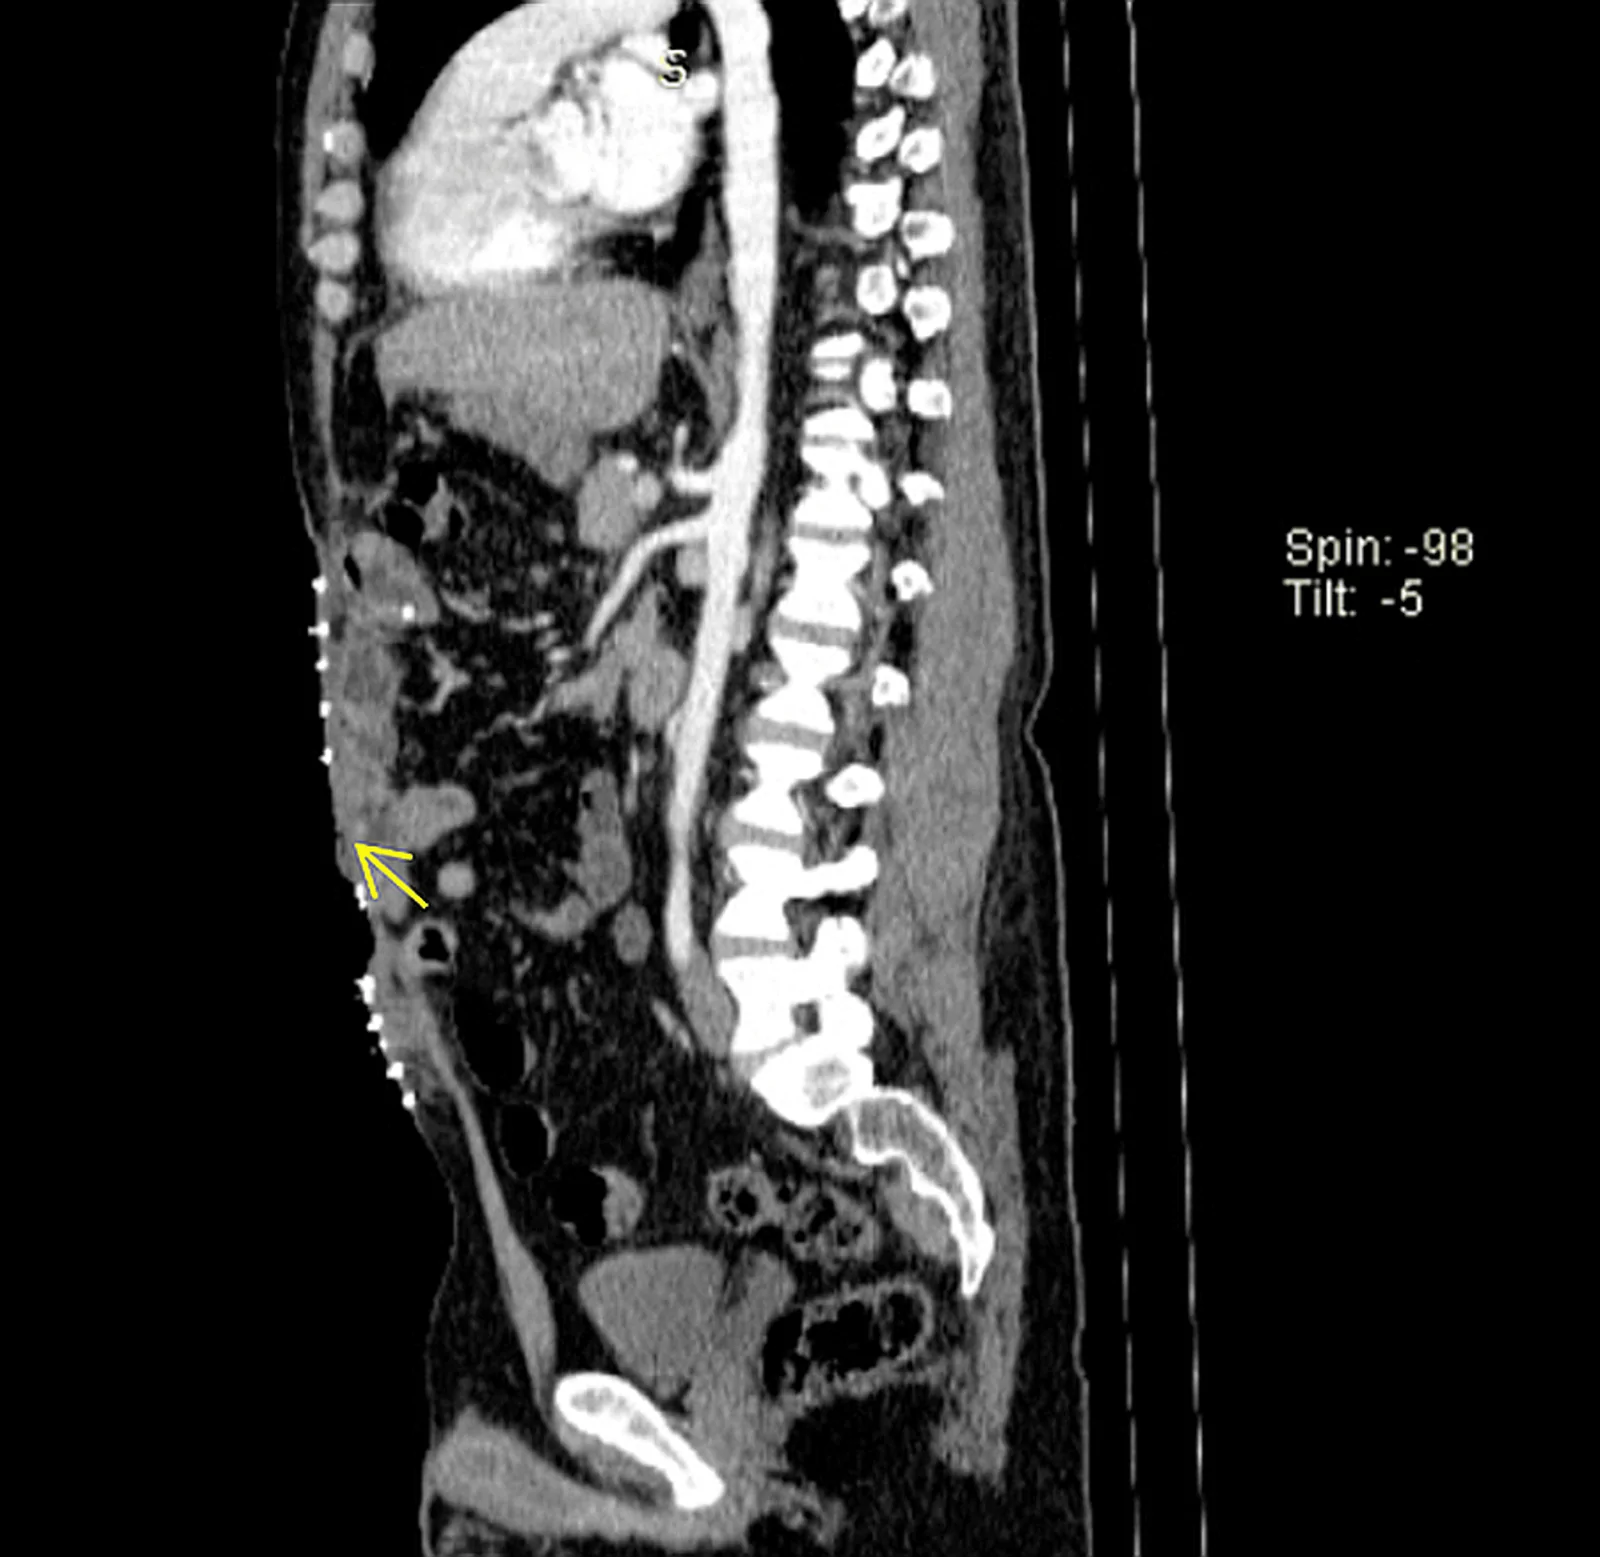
Sagittal computed tomography image showing the craniocaudal view of the hernia without radiological evidence of loss of domain. The arrow indicates the precise location of the hernia
